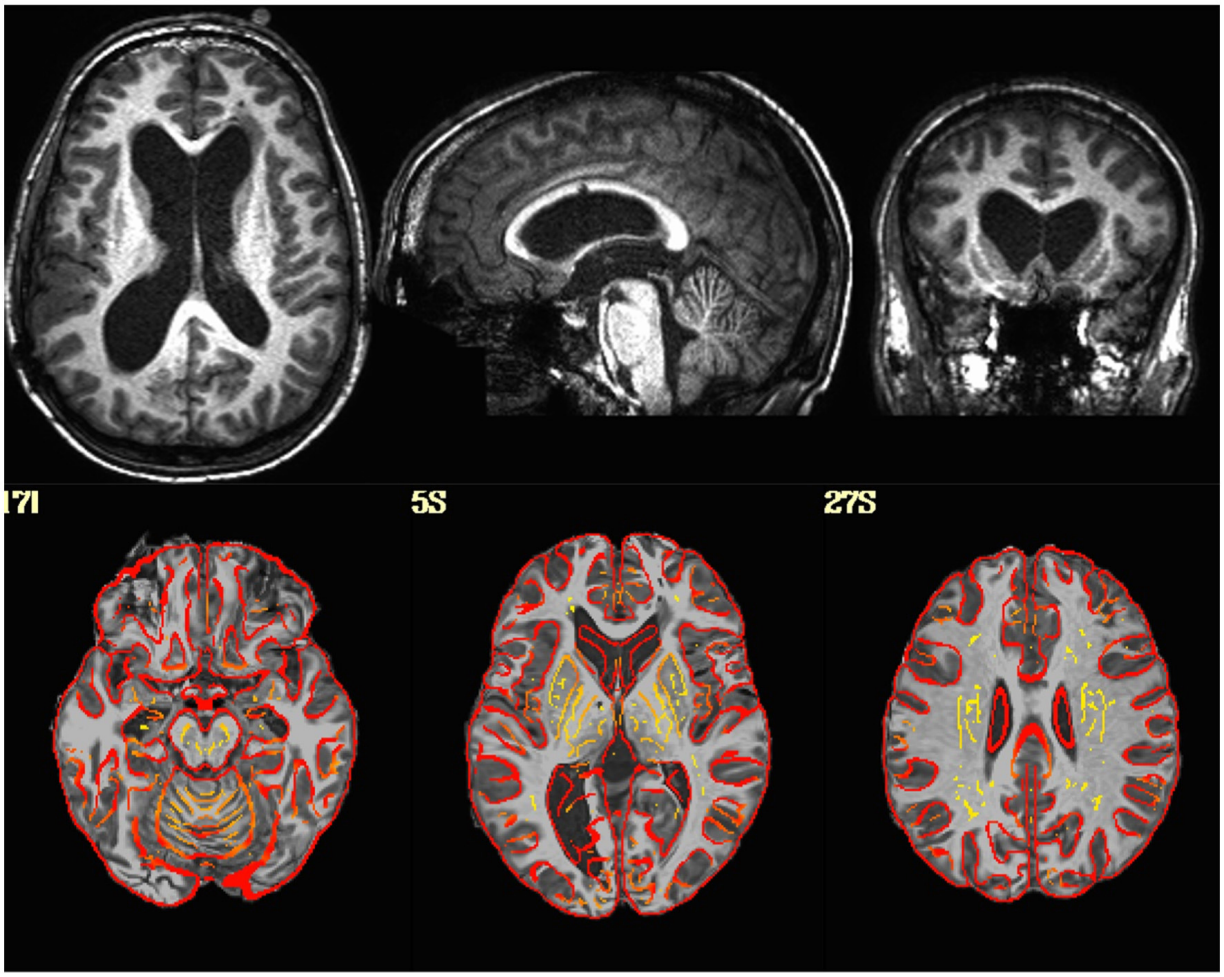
(Top Row) T1-weighted image in native space for a subject with enlarged ventricles. (Bottom Row) T1-weighted image non-linearly aligned to template space as underlay, with the gray/white matter boundaries from the template brain overlayed in red. Dice coefficient between the subject's T1 and the template = 0.96.
